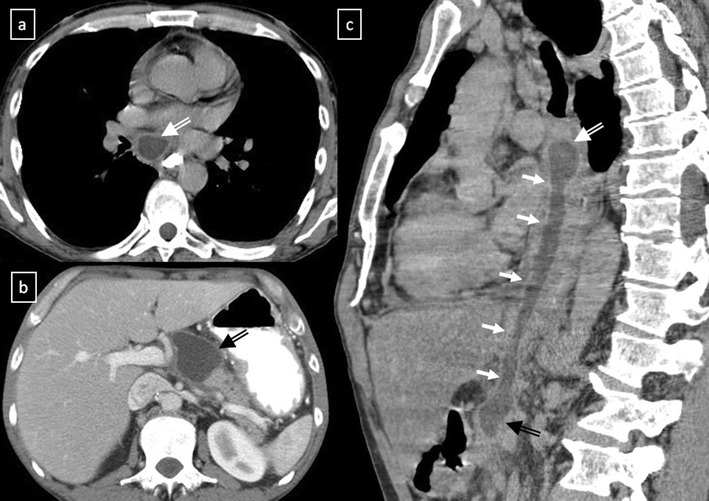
Pancreatic pseudocyst in a 52-year-old man with a recurrent pancreatitis history. Axial (a, b) and oblique-sagittal multiplanar reconstruction (c) CT scan show a thin-walled peri-oesophageal fluid-lesion (*white open arrows*) that comunicates with an intrapancreatic fluid-collection (*black open arrows*) through oesophageal hiatus by a duct fistula (*white arrows*)
